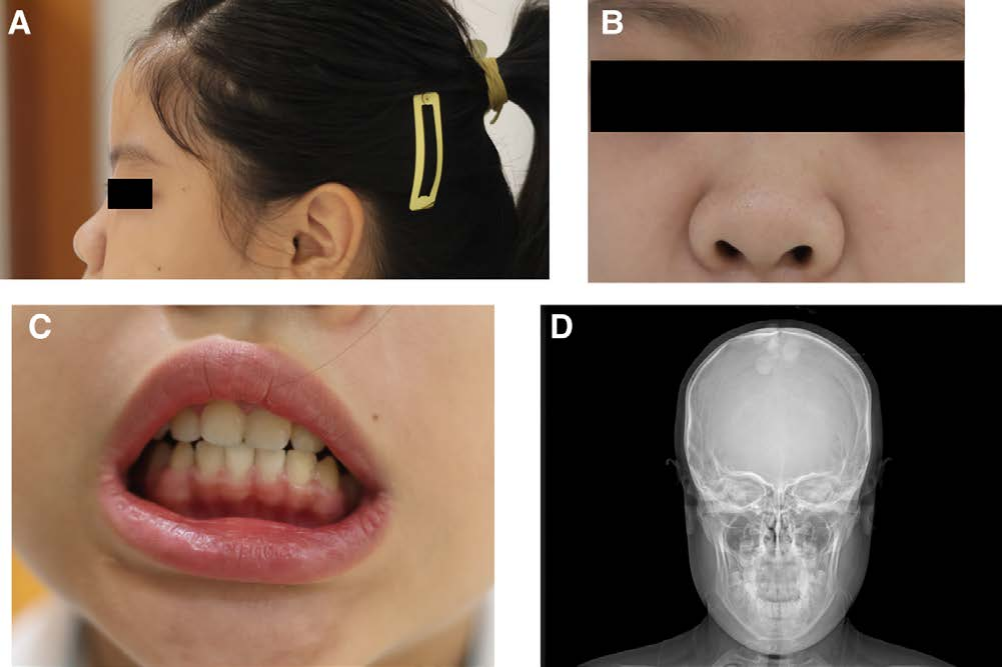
Craniofacial features of the patient. (A–C) Distinctive facial appearance, characterized by flat forehead, hypertelorism, epicanthal folds, gingival enlargement, thick nasal wings, flat nasal tip, and a flat and broad nasal bridge. Black bars covering the eyes in (A, B) are used to protect the patient privacy. (D) X-ray scan showing micrognathia, maxillary hypoplasia, and slightly widened cranial suture.
